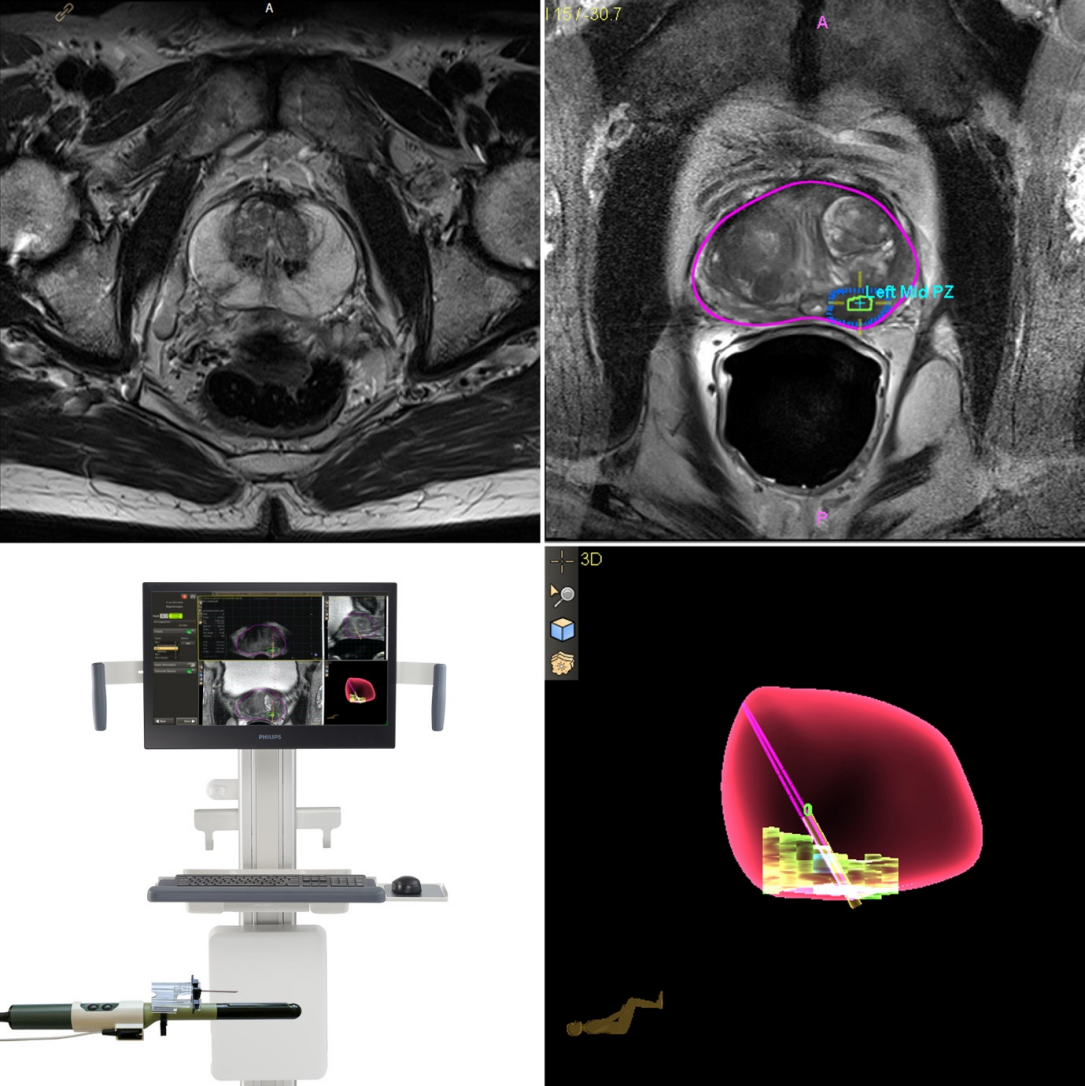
Example MRI fusion transperineal prostate biopsy using in-line needle guidance under local anaesthetic (UronavTM, Philips): Prostate MRI (top right) with contoured prostate (pink) and lesion (top left; green/blue); the needle guide is mounted to the probe (bottom left) to allow needle tracking within the sagittal plain; 3D animated documentation of the prostate, lesion and current plain.
